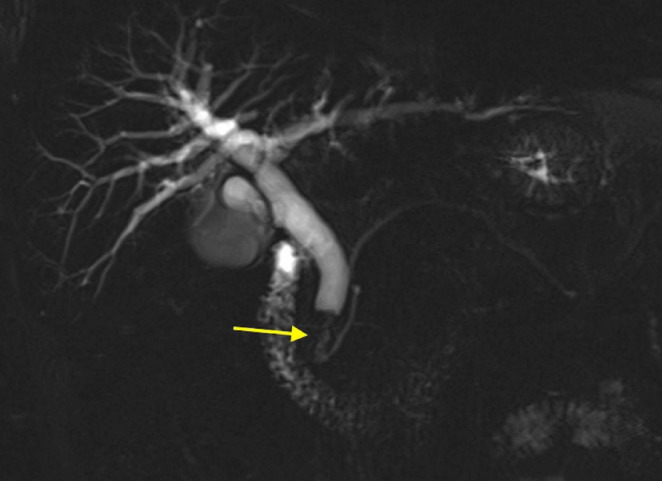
Coronal T2 SPACE MRI confirming distal CBD stricture as indicated by the arrow, with upstream biliary tree dilatation. Normal calibre main pancreatic duct suggests the mass originates within CBD rather than from the pancreas or ampulla
